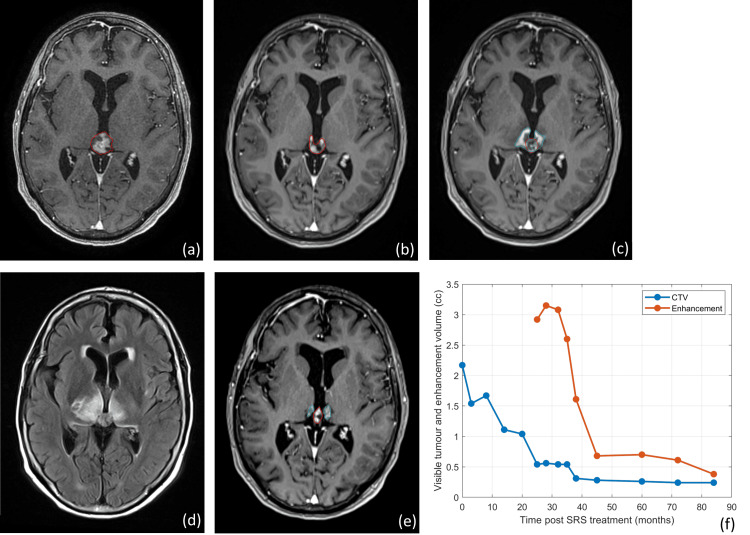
T1 post-gadolinium magnetic resonance imagings (MRIs) at the time of treatment (a), 20 months post-treatment (b), 25 months post-treatment (c), 25 months post-treatment flair MRI (d), and 84 months post-treatment T1 (e). The pineal parenchymal tumor of intermediate differentiation (PPTID) clinical target volume (CTV) as detected at the given time point are shown on each T1 image in red, as well as the enhancement region detected starting at 25 months post-treatment in blue. The CTV volume and enhancement region volume at each time point since it developed are plotted in panel (f).
